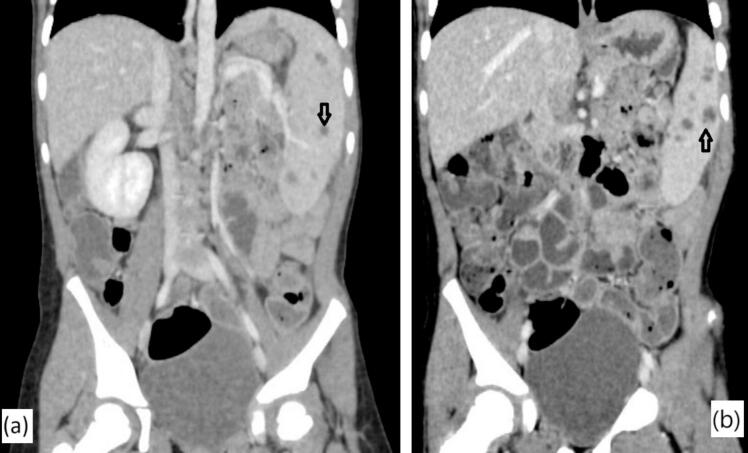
a, b: Contrast-enhanced computed tomography of the abdomen (Coronal Sections) showing mild splenomegaly with multiple hypodense splenic lesions (black arrow).
